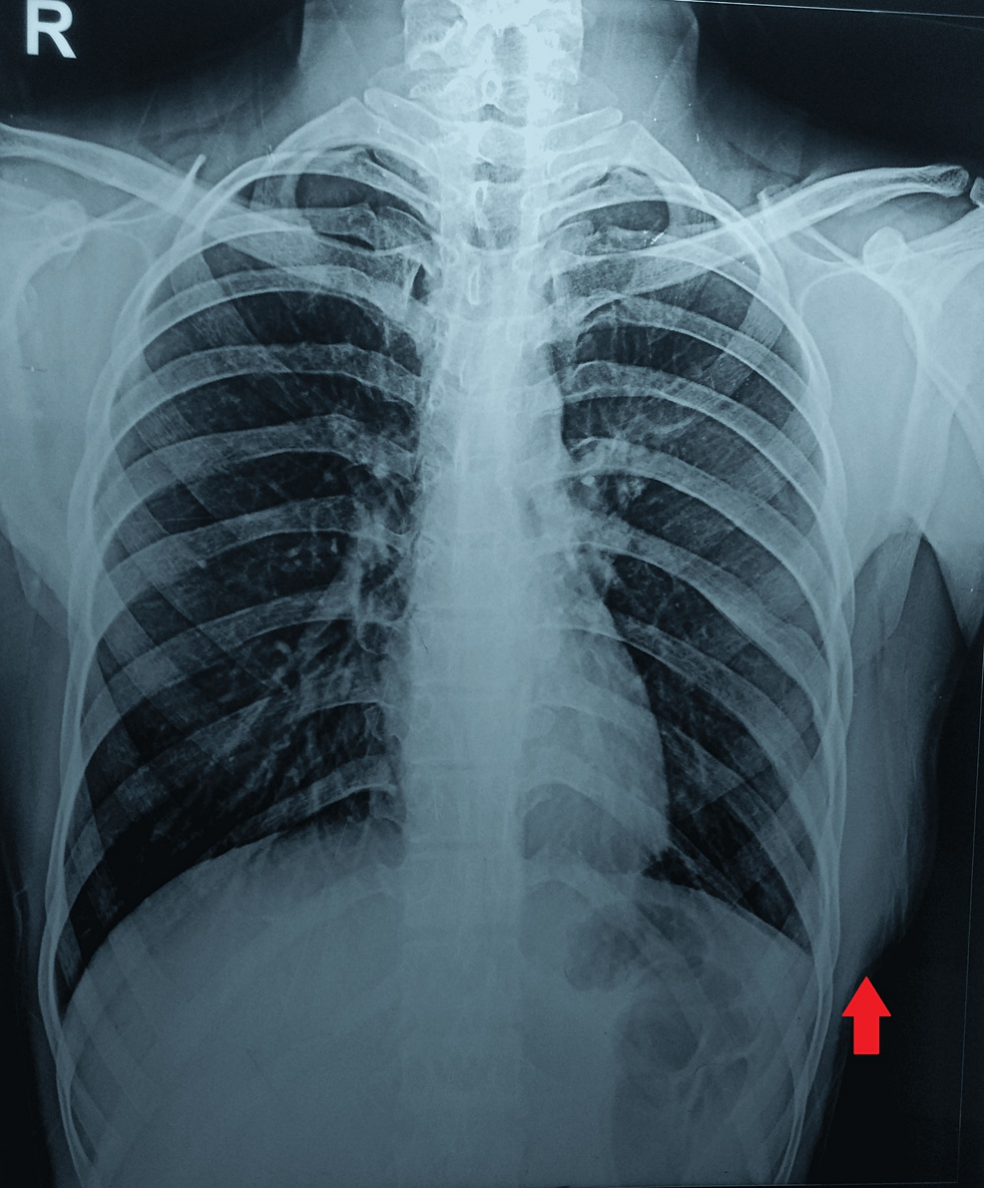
A plain chest radiograph The radiograph showed no pulmonary involvement but indicated the presence of soft tissue swelling.
